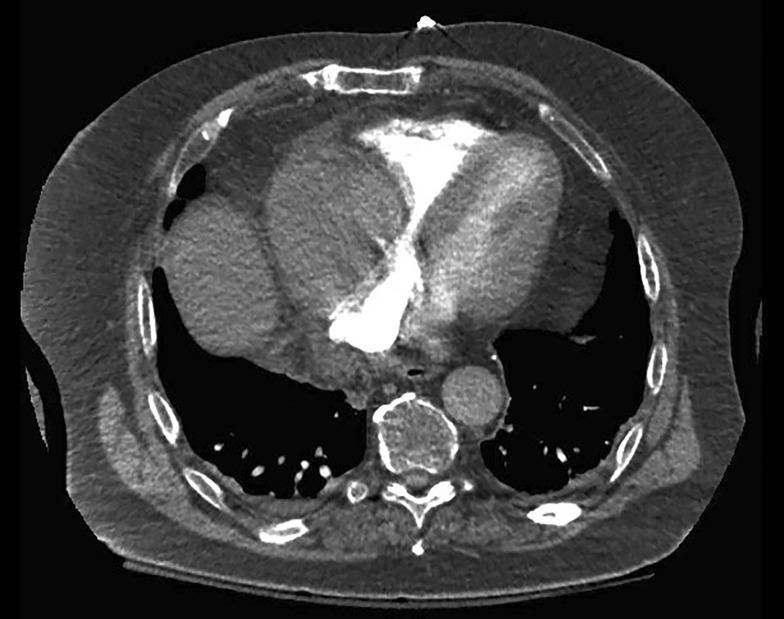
The computed tomography image at presentation
